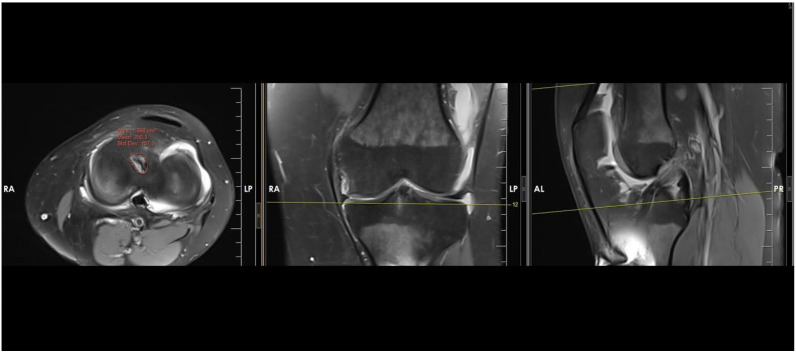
The tibial tunnel aperture was analyzed on the joint surfaces of the tibia post-anterior cruciate ligament reconstruction. The tunnel aperture area at the joint interface was precisely measured using reference lines and a freehand measurement tool for accuracy (Uppercase letters and yellow lines are automatically generated by the MRI analysis software and represent measurement values and reference boundaries).
